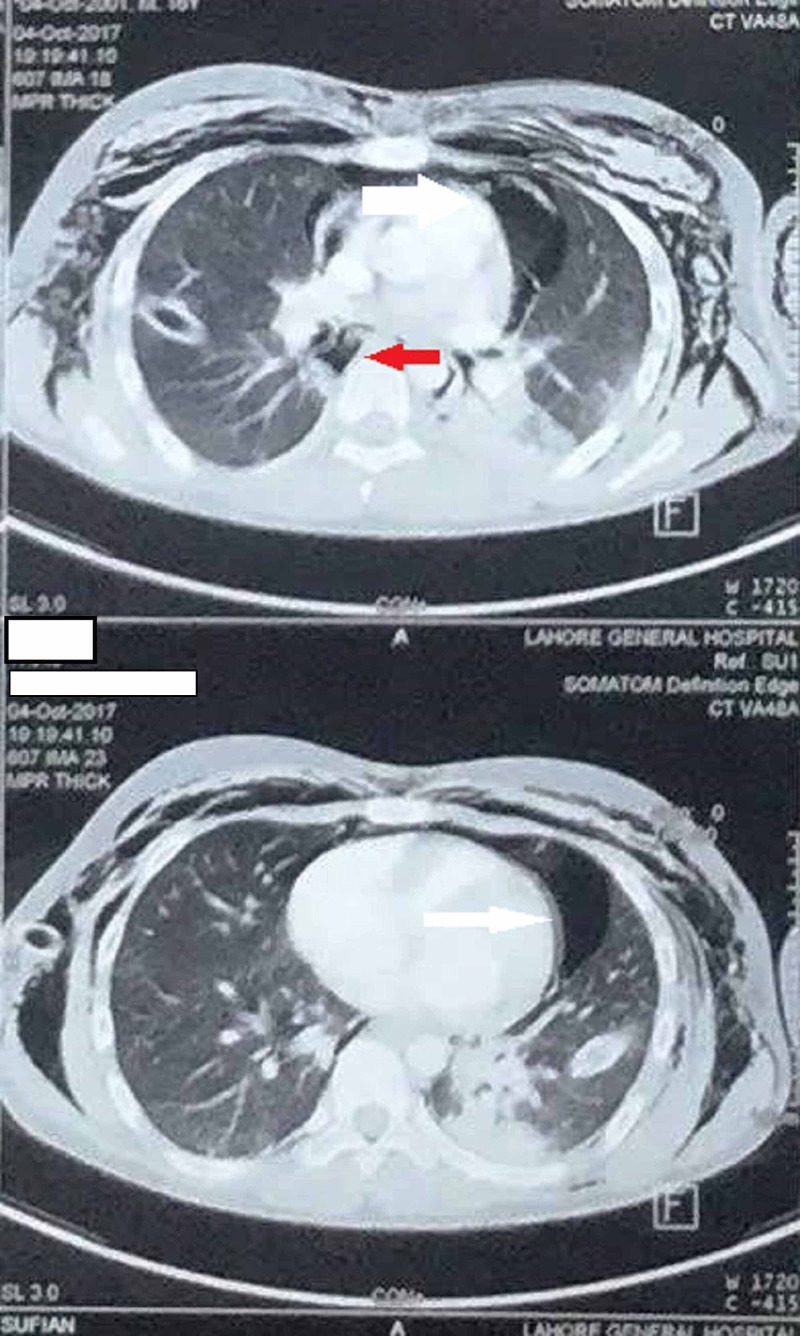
HRCT of the chest showing pneumothorax (white arrow) and pneumomediastinum (red arrow) HRCT - high-resolution CT
